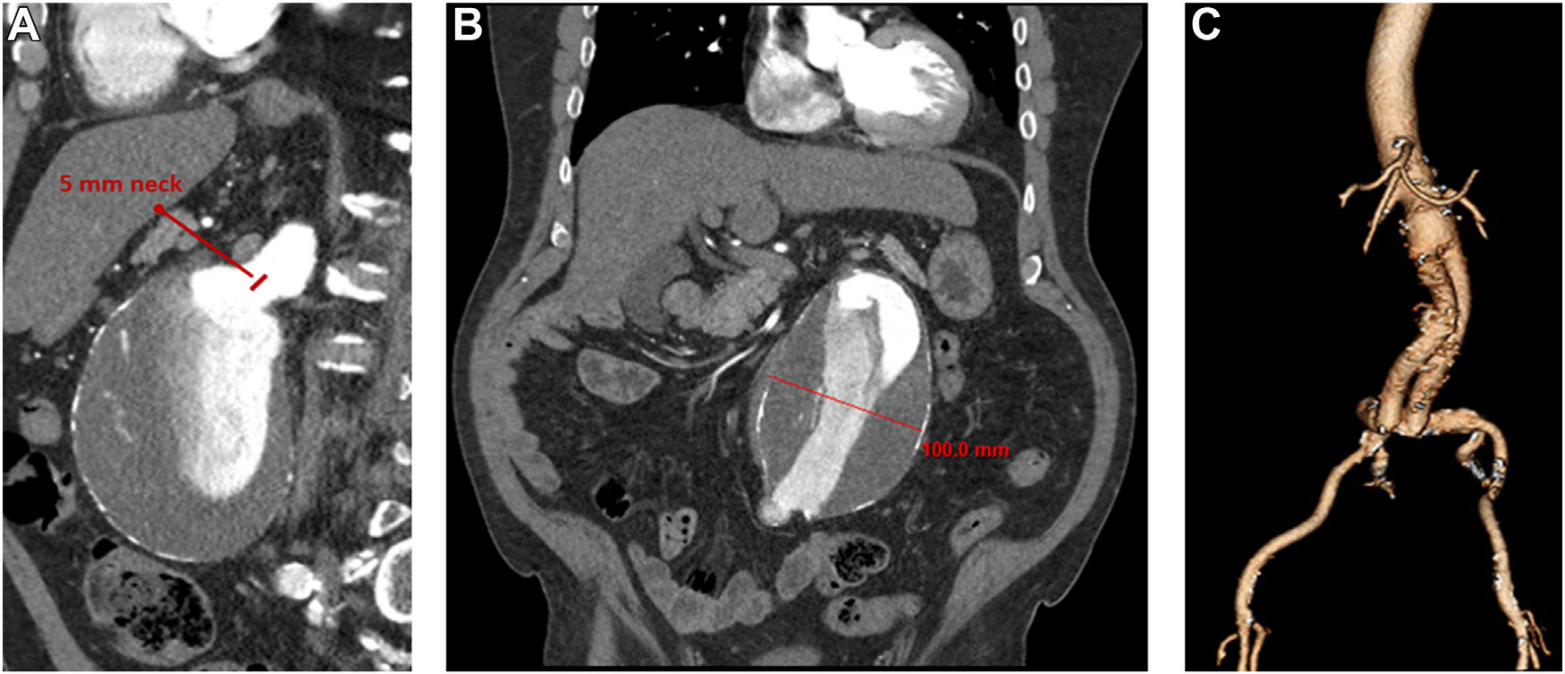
A, Sagittal computed tomography view demonstrating a short, 5-mm-long, angulated abdominal aortic aneurysm neck. B, Coronal view showing the maximal diameter of the aneurysm was 100 mm. C, Three-dimensional computed tomography scan reconstruction at 1 year postoperatively demonstrating widely patent anastomoses.
